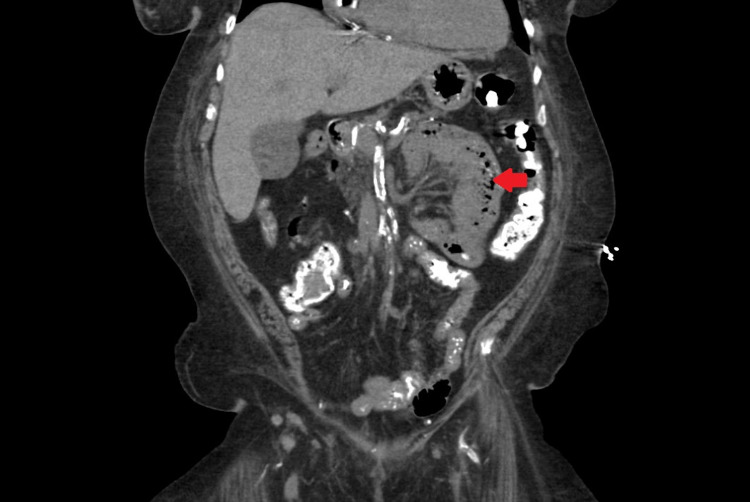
CT scan of abdomen and pelvis; red arrow points to small bowel pneumatosis.
